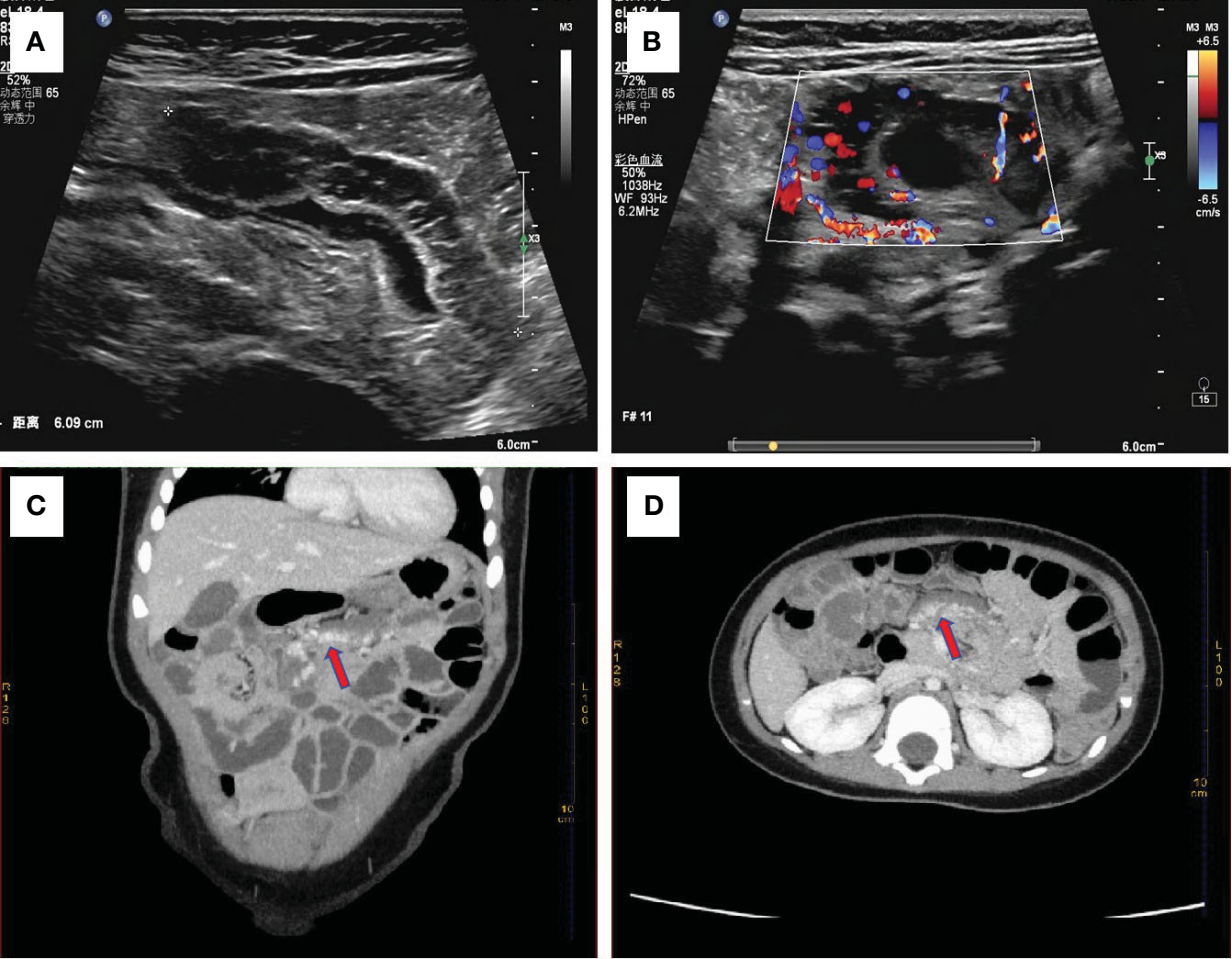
(A) Abdominal color ultrasonography shows stiff morphology of small intestine with slightly obstructed passage of contents, suggesting segmental venous malformation. (B) Abundant blood flow signals are seen around the wall of the small intestine. Enhanced abdominal CT shows focal thickening of the small intestine wall with tortuous, chaotic and high-density vascular shadows. The blood supply artery is from the superior mesenteric artery. This indicated venous malformation of the small intestine (C) with coronary vascular signs and (D) with cross-sectional vascular signs.
